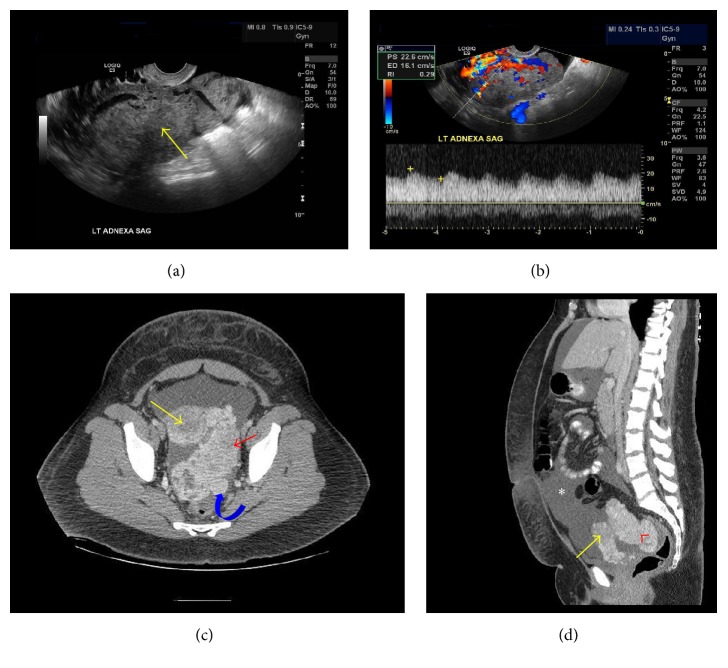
(a) Sagittal transvaginal ultrasound image: large heterogeneous adnexal mass. (b) Sagittal pelvic US with Doppler imaging: vascular adnexal mass with low resistant arterial flow (RI = 0.29). (c) Axial CT pelvis with IV contrast: yellow arrow: uterus, red arrowhead: enhancing adnexal mass, and curved blue arrow: cul-de-sac metastasis. (d) Sagittal CT pelvis with IV contrast: yellow arrow: uterus, red arrowhead: adnexal mass, and white asterisk: ascites.
